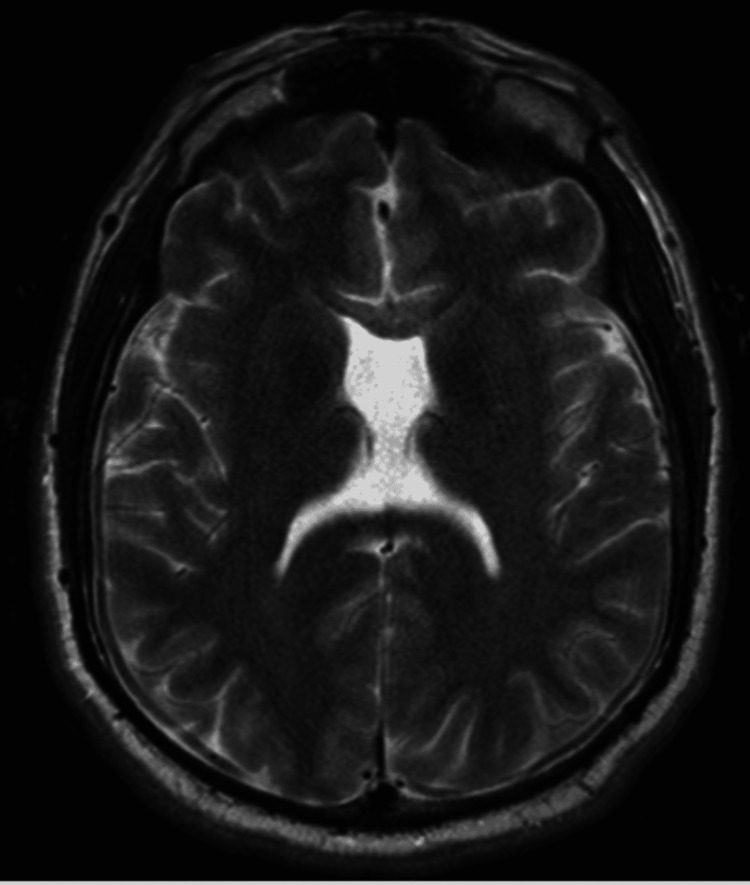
MRI demonstrating absent nasal septum possibly secondary to holoprosencephaly
